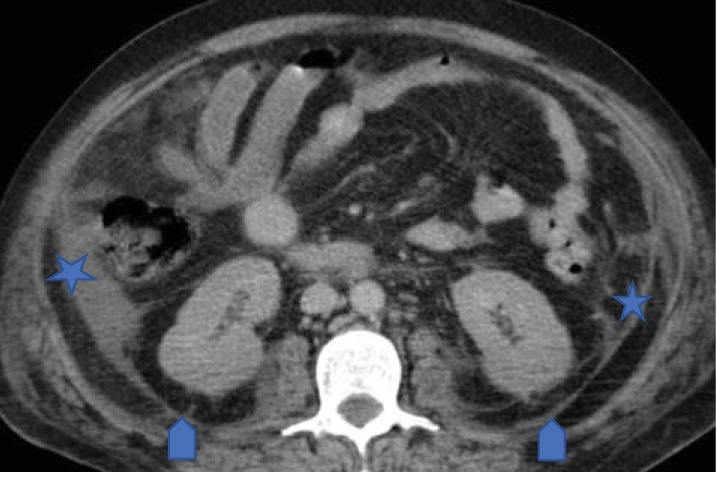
Axial CT image of the abdomen through the level of the kidneys showing thickening of posterior pararenal fascia (arrows) and lateral conal fascia (asterisk).
